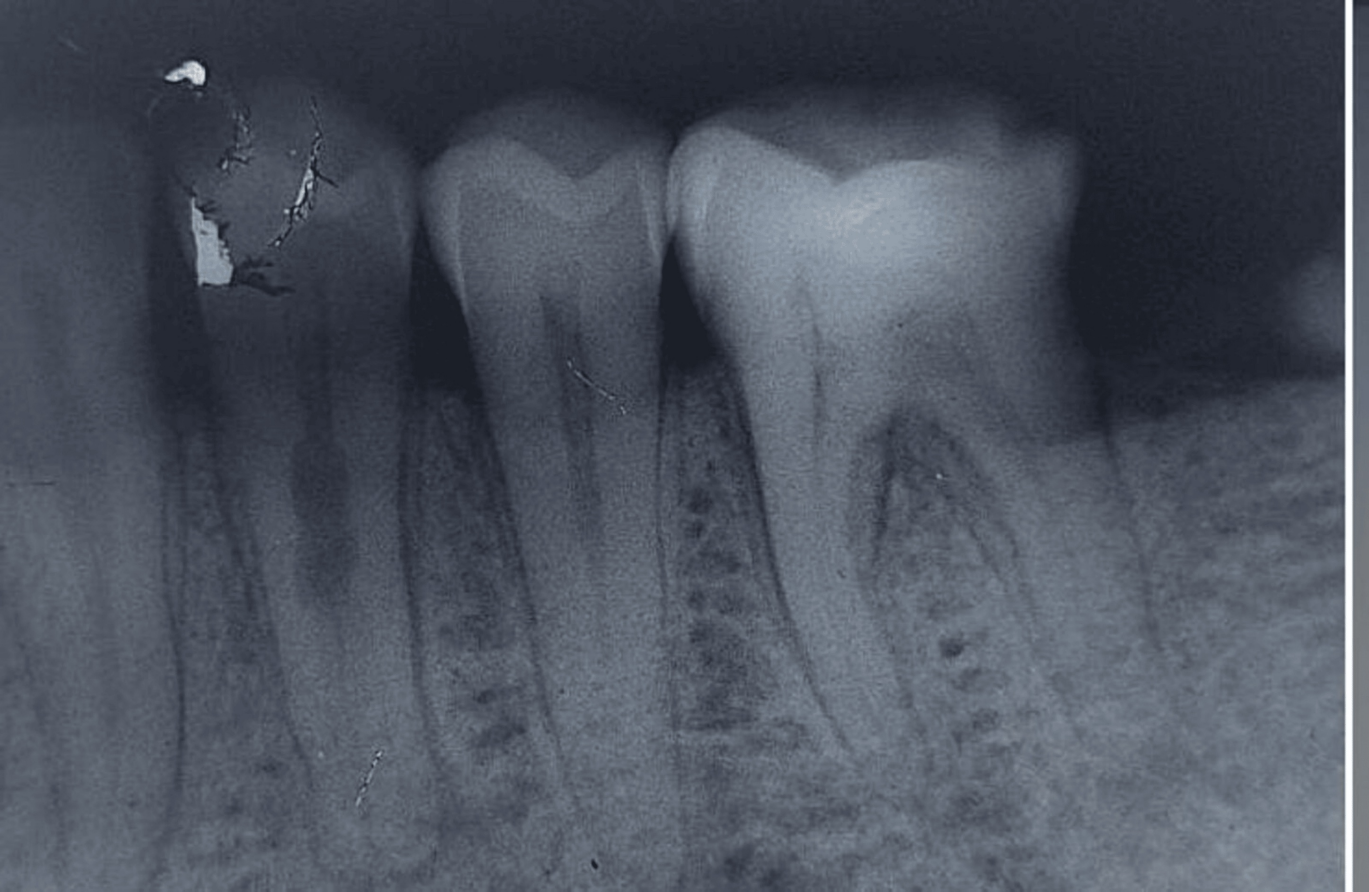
Pre-operative intra-oral periapical radiograph of the mandibular left first premolar in the patient in the case study, showing an unusual anatomy of two roots, and widening of the apical periodontium
